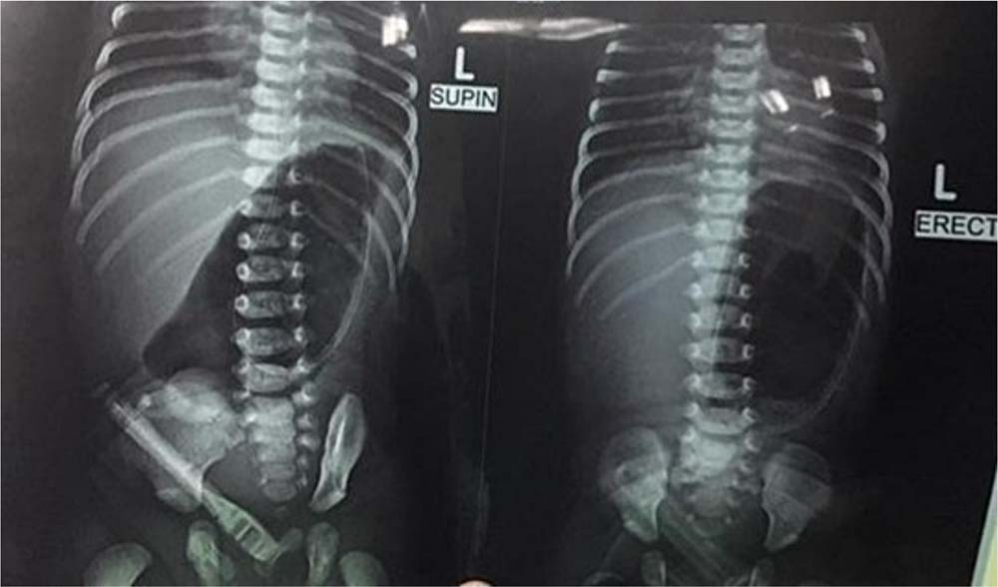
Plain abdominal X-ray showing gastric outlet obstruction.
